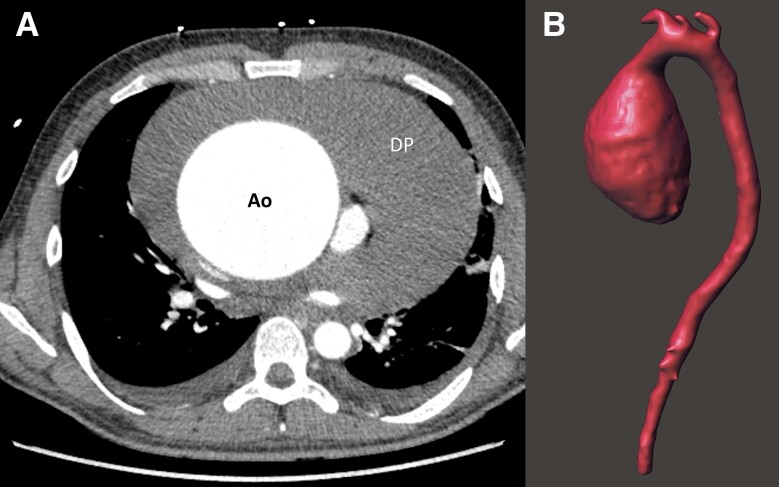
(*A*) Contrast-enhanced axial computed tomography (CT) reveals an aneurysmal ascending aorta (ao) along with a severe pericardial effusion (PE). (*B*) Isovolumetric reconstruction of the entire aorta displays the distinctive ‘sausage-like’ morphology of the ascending aortic aneurysm, while the remainder of the aorta appears to be of normal size.
